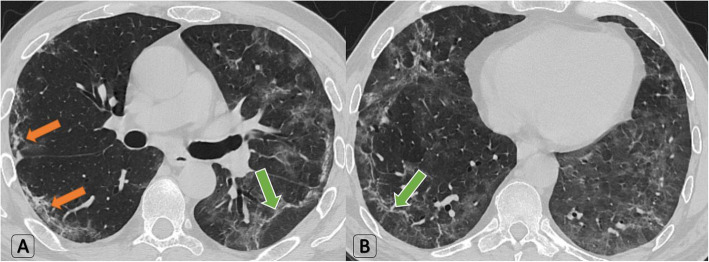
A 37-year-old male patient known for COVID-19: Follow-up HRCT scans performed 18 days after announcing recovery. Patient is completely asymptomatic. O_2_ saturation = 95%. Unremarkable laboratory results. a-b Axial HRCT lung window showed residual bilateral large GGOs (approximating 50% of lobar volume), atoll sign (green arrows), and curvilinear fibrotic bands (orange arrows). Clinical decision was no need for re-isolation or re-hospitalization
